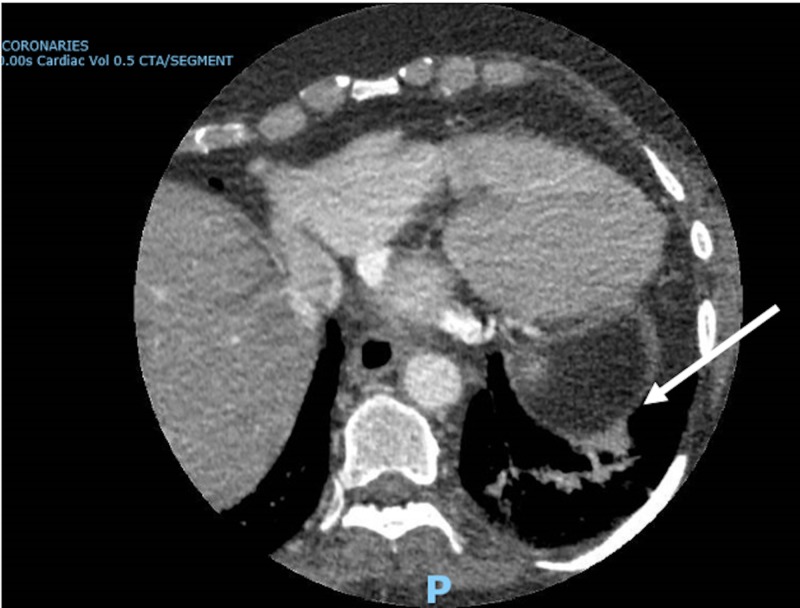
Computed tomography coronaries showing a large, multilobed left atrial appendage without evidence of thrombus (white arrow).
